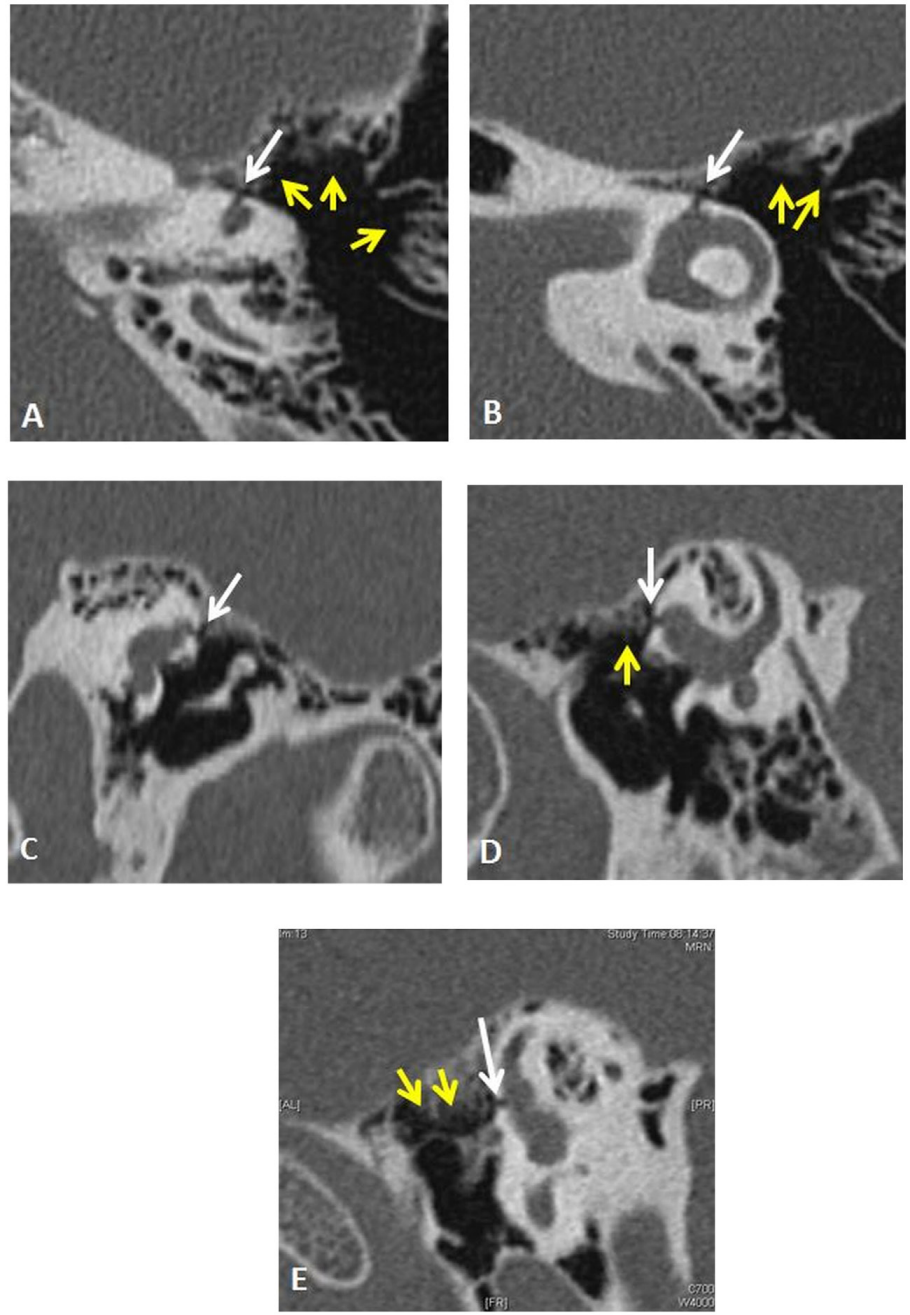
Left temporal bone high-resolution computed tomography (HRCT) demonstrates by its shape and topography (white arrows) an original ampullar dehiscence of the superior semicircular canal. The infra-millimetric (~0.5 mm) and tubular in shape dehiscence was disposed between the ampullaes of the lateral and superior semicircular canal and the medial epitympanic wall. An important pneumatization of perilabyrinthine air cells was observed [yellow arrows (A,B,D,E)]. (A,B) axial left temporal CT scan. (C) Reformatted image of the left labyrinth (oblique coronal). (D) Reformatted image of the left labyrinth (oblique sagittal). (E) Reformatted image of the left labyrinth (oblique sagittal). The ampullar dehiscence on the left superior semicircular canal’s anterior crus was visible on two perpendicular planes [axial (A,B), coronal plane (C), Pöschl plane (D), and in oblique sagittal plane (E)]. Its diameter was measured between 0.4 and 0.5 mm.
